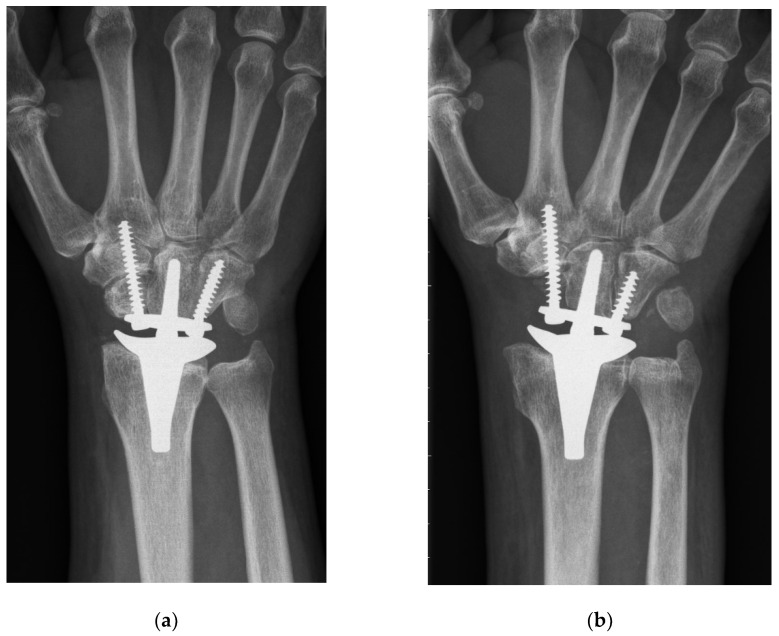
Postoperative radiograph showing both applied implantation techniques: ReMotion implantation using (a) CCR (conventional carpal resection) and (b) PRC (proximal row carpectomy). The results merely differ in the distal pole of the scaphoid being removed in the PRC cohort.
